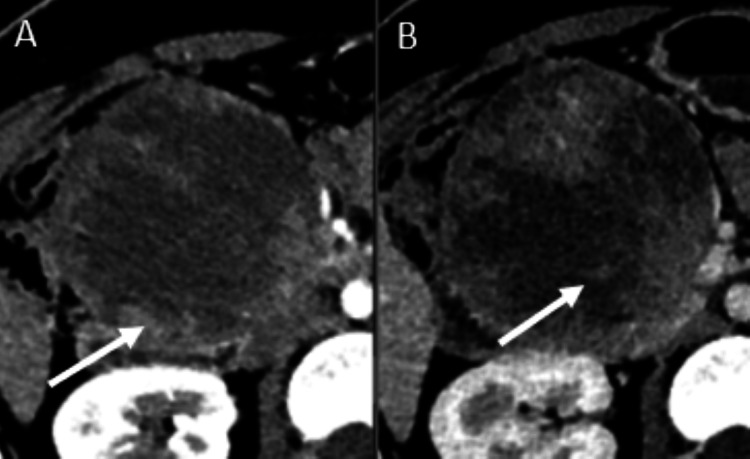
Contrast-enhanced CT demonstrating a pancreatic solid pseudopapillary neoplasm (A) Axial CT image showing a well-defined mass with peripheral enhancement (arrow) suggestive of a peripheral enhancing capsule; (B) The lesion demonstrates central hypodense areas (arrow) consistent with necrosis or cystic degeneration.
